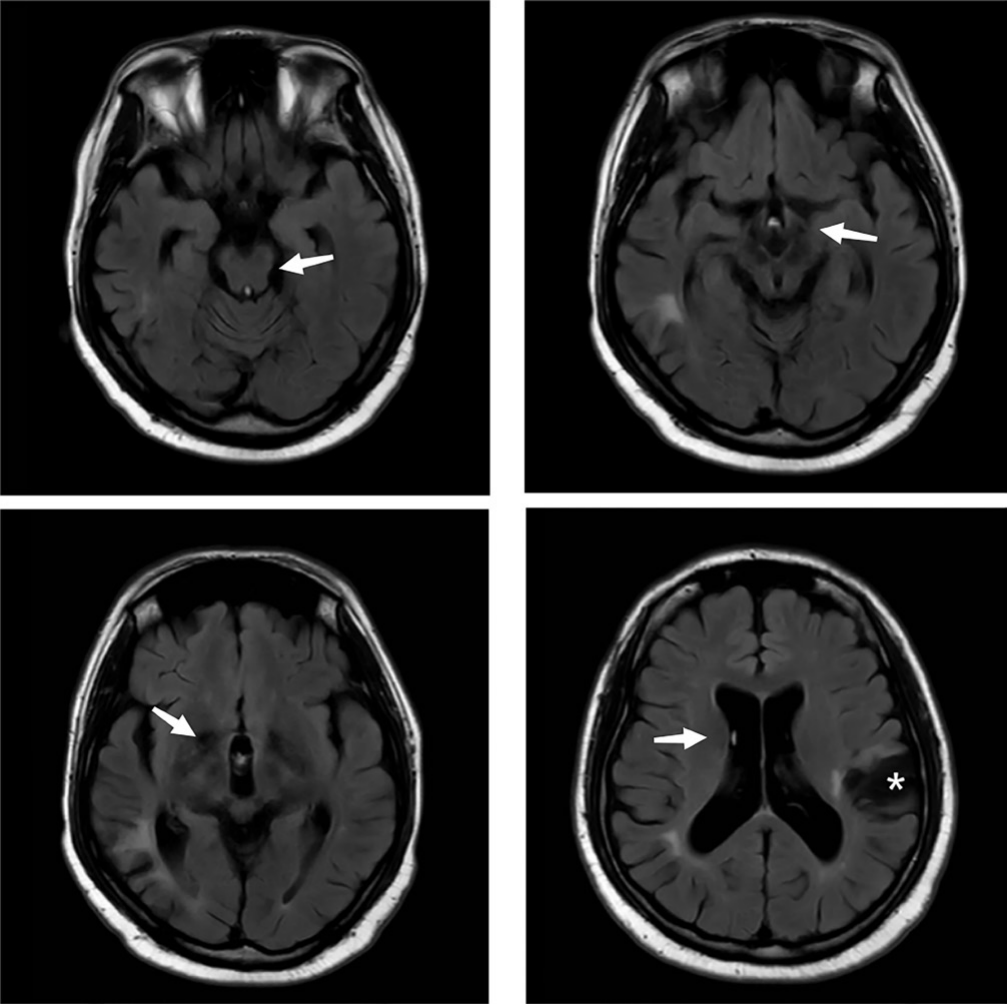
Cranial MRI 6 months after treatment of high-dose glucocorticoids: Most abnormal signals in the bilateral basal ganglia and midbrain disappeared (arrows). *obsolete cerebral infarction. MRI, magnetic resonance imaging.
